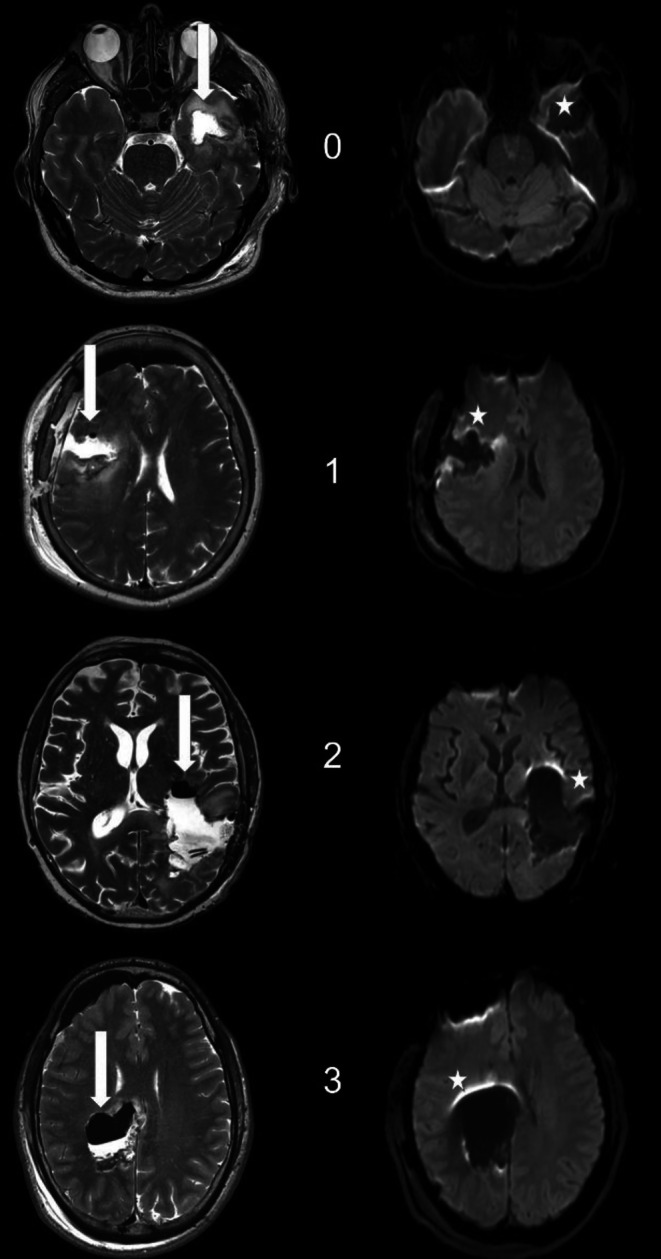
Susceptibility (air) artifacts rating scale (*left* T2-weighted turbo spin-echo images, *right* transversal echo-planar diffusion weighted images, EPI DWI, with b = 1000 s/mm^2^, *arrows* resection area with or without T2-hypointense air, *stars* associated area with or without air artifacts). Air artifacts rating (in the resection area): 0 no air artifacts, 1 small artifacts (e.g. small air bubbles) not affecting more than 5% of the brain tissue, 2 moderate artifacts affecting less than 25% of the neighboring brain tissue, 3 severe artifacts affecting more than 25% of the neighboring brain tissue
